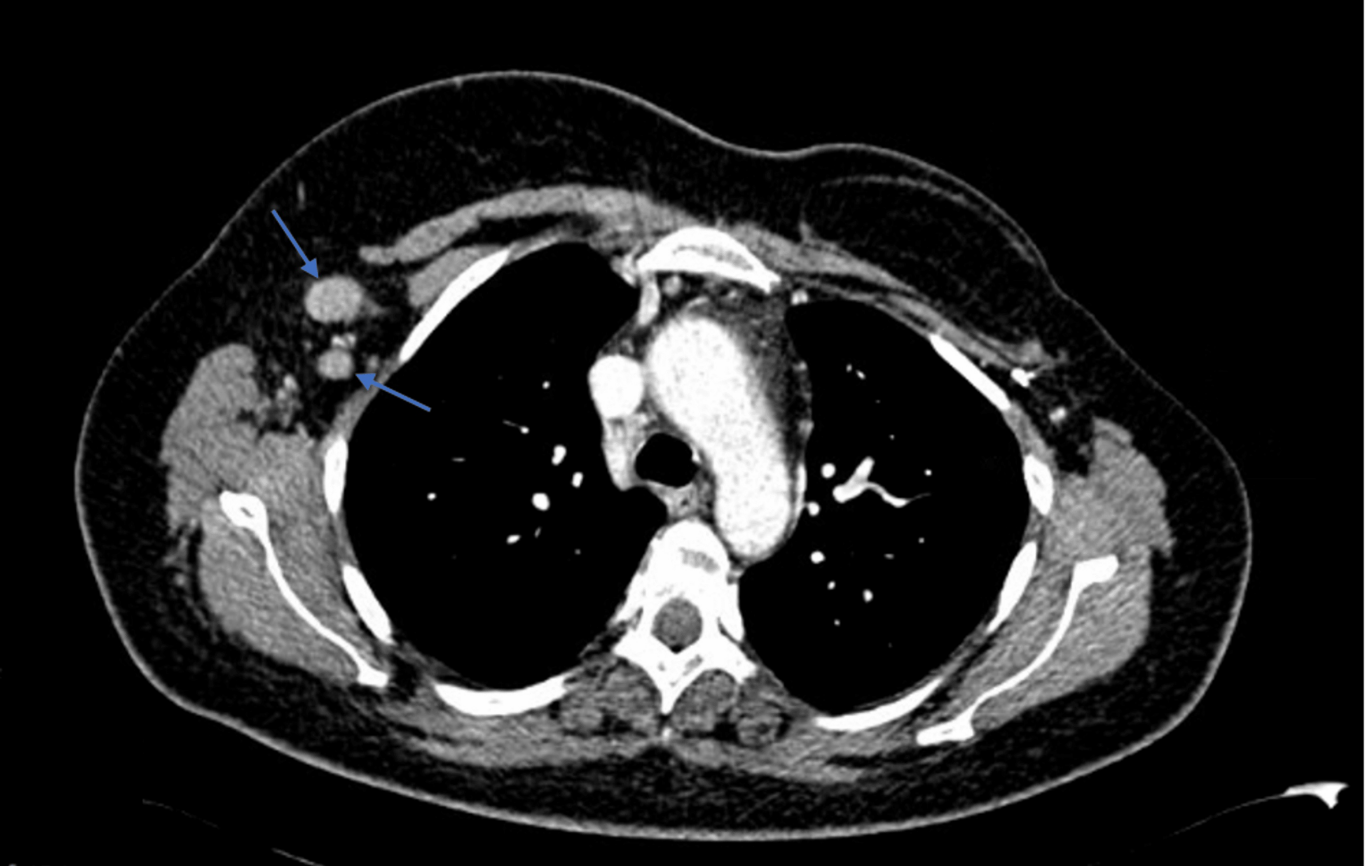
Initial CT showing adenopathies in the right axillary region (arrows). CT: Computed tomography
